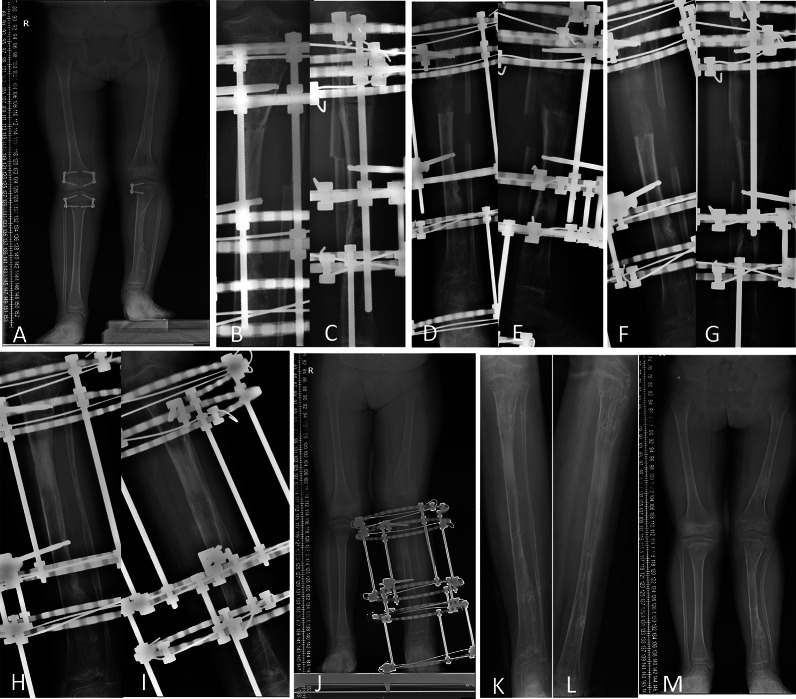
An 8Y11M girl (patient 6) with Ollier’s disease, left tibia lengthened by 6.65 cm, 6.4°AC, with Illizarov fixator. A Preoperative full length AP radiograph. B, C, AP and lateral radiographs during operation. D, E AP and lateral radiographs after 38 days of lengthening. F, G AP and lateral radiographs at the end of lengthening after 68 days. H, I, AP and lateral radiographs before removal of the external fixator, 7.5 months after operation. J Full-length AP radiograph before removal of the external fixator, 8 months after operation. K, L AP and lateral radiographs after removal of the external fixator, 8 months after operation. M Full-length AP radiograph 6 months after removal of the external fixator
